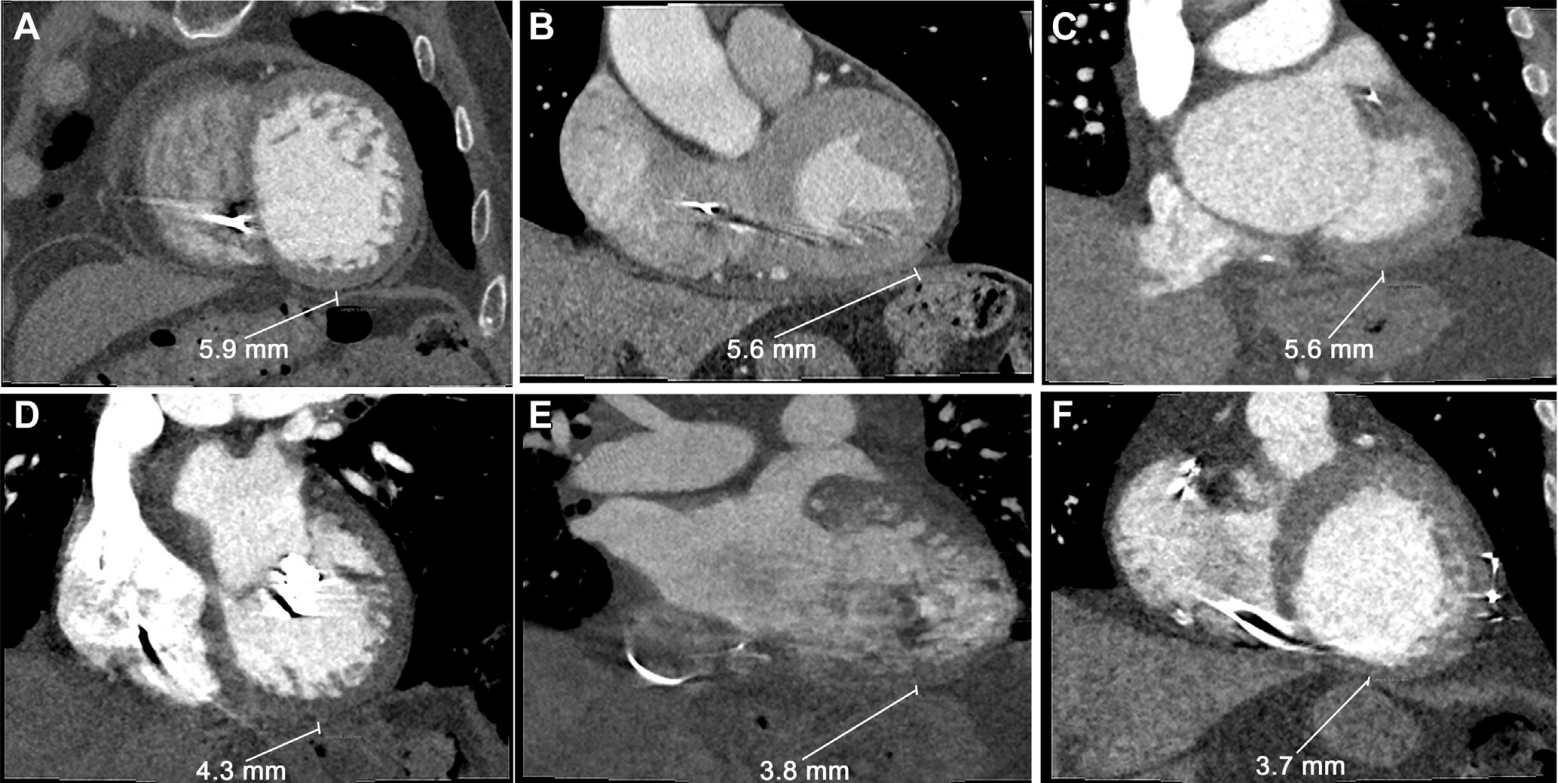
Close proximity of the inferior wall to the stomach. Computed tomography images from all patients showing the close proximity of the stomach to the basal inferior left ventricle wall (4.8 ± 1.0 mm) for each study patient. The smallest distance was 3.7 mm in patient 6.
